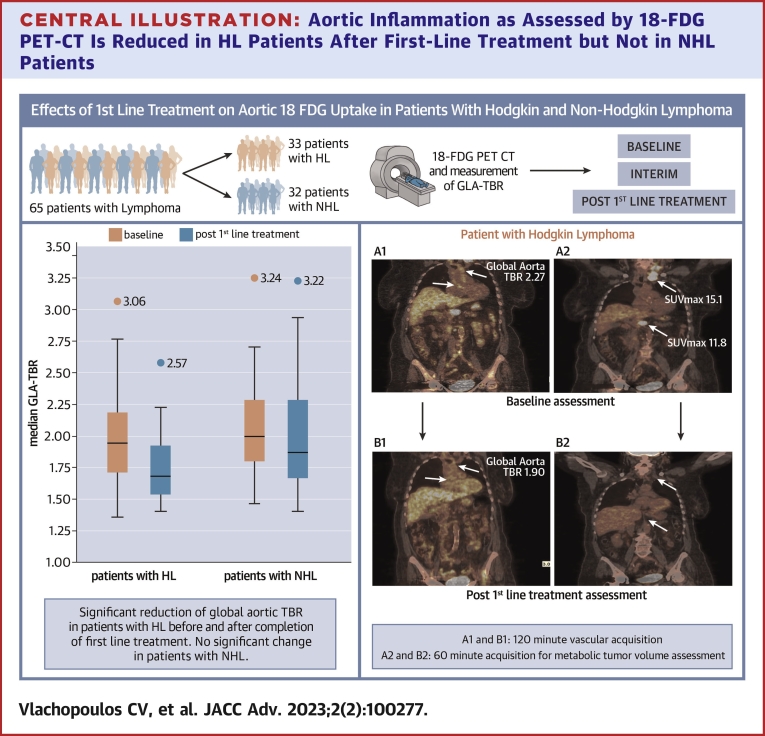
Aortic Inflammation as Assessed by 18-FDG PET-CT Is Reduced in HL Patients After First-Line Treatment but Not in NHL Patients These findings imply that different pathophysiological pathways and different therapies might affect the arterial bed in different ways for patients with lymphoma. GLA-TBR = global aortic target-to-background ratio; HL = Hodgkin lymphoma; NHL = non-Hodgkin lymphoma; SUVmax = standard uptake value max.
